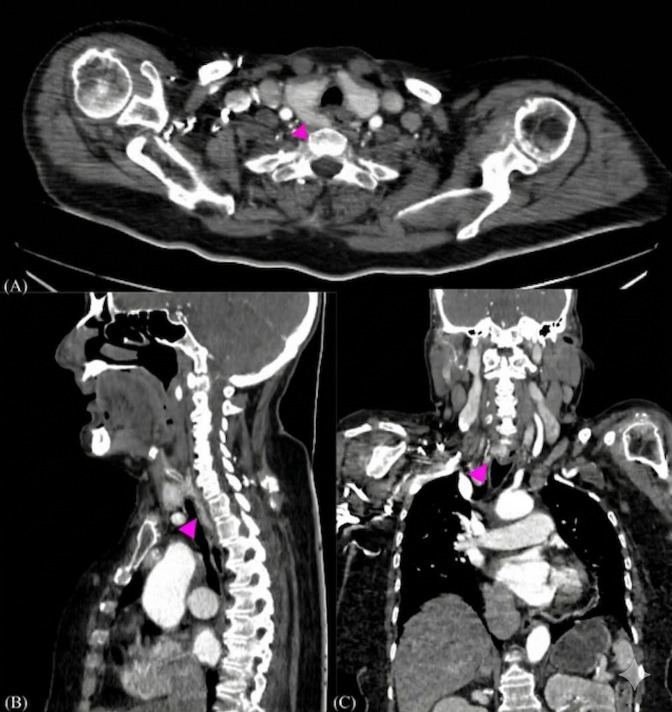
Contrast-enhanced computed tomography of the right thyroid lesion (purple pointer). (A) Transverse view. (B) Sagittal view. (C) Coronal view.
